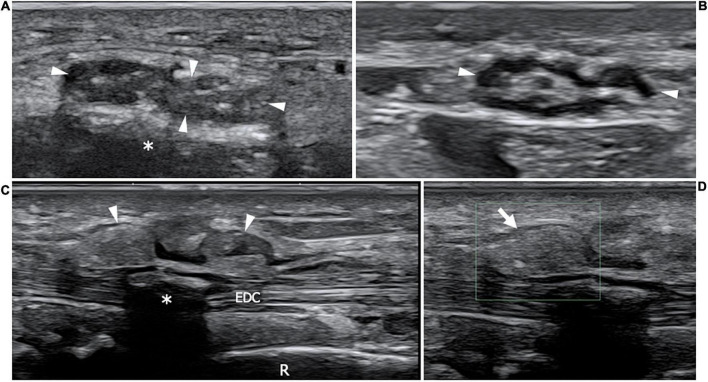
This figure shows two 22 MHz HRUS images (A,B) and two 18 MHz HRUS images (C,D) of a 62 years old male affected by multiple myeloma who developed an AL amyloid arthropathy. (A) An axial view of the median nerve (white arrowheads), situated in the proximity of the flexor retinaculum is presented. The nerve appears enlarged, with clumped hypoechoic fascicles, a finding which is related to the compression due to the underlying amorphous, hyperechoic material (white asterisk); the nerve echotextural alterations become even more evident if the image is compared to the image in (B) where the median nerve (white arrowheads) is visualized in the short axis at a more cranial level in the forearm. (C) On the dorsal aspect of the same wrist, a huge distension of the extensor digitorum communis tendons (EDC) synovial sheath (white arrowheads) can be observed. At the Eco-Color Doppler evaluation showed in (D), the inhomogeneous, hyperechoic material distending the tenosynovial sheath (white arrow) results avascular, a finding which is compatible with an intrasynovial amyloid deposition. White asterisk, intra-tendon calcification; R, radius.
